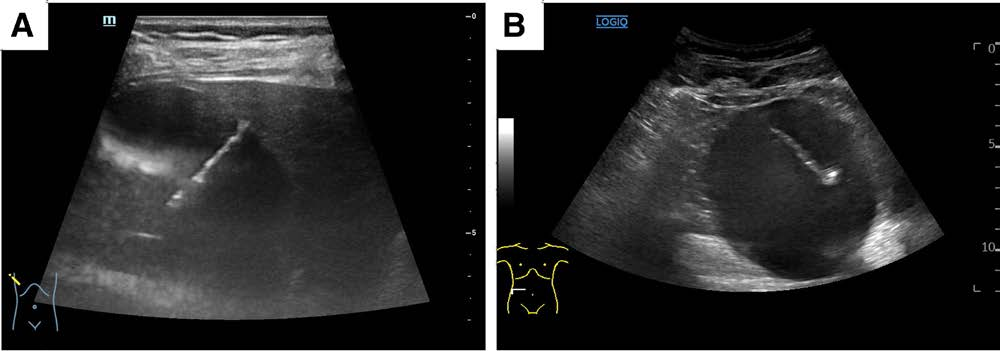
US-guided tube drainage for giant hepatic and renal cyst. (A) A drain is placed into the cyst via puncture of normal liver tissue. (B) US-guided placement of drainage tubes in renal cyst. US = ultrasound.
